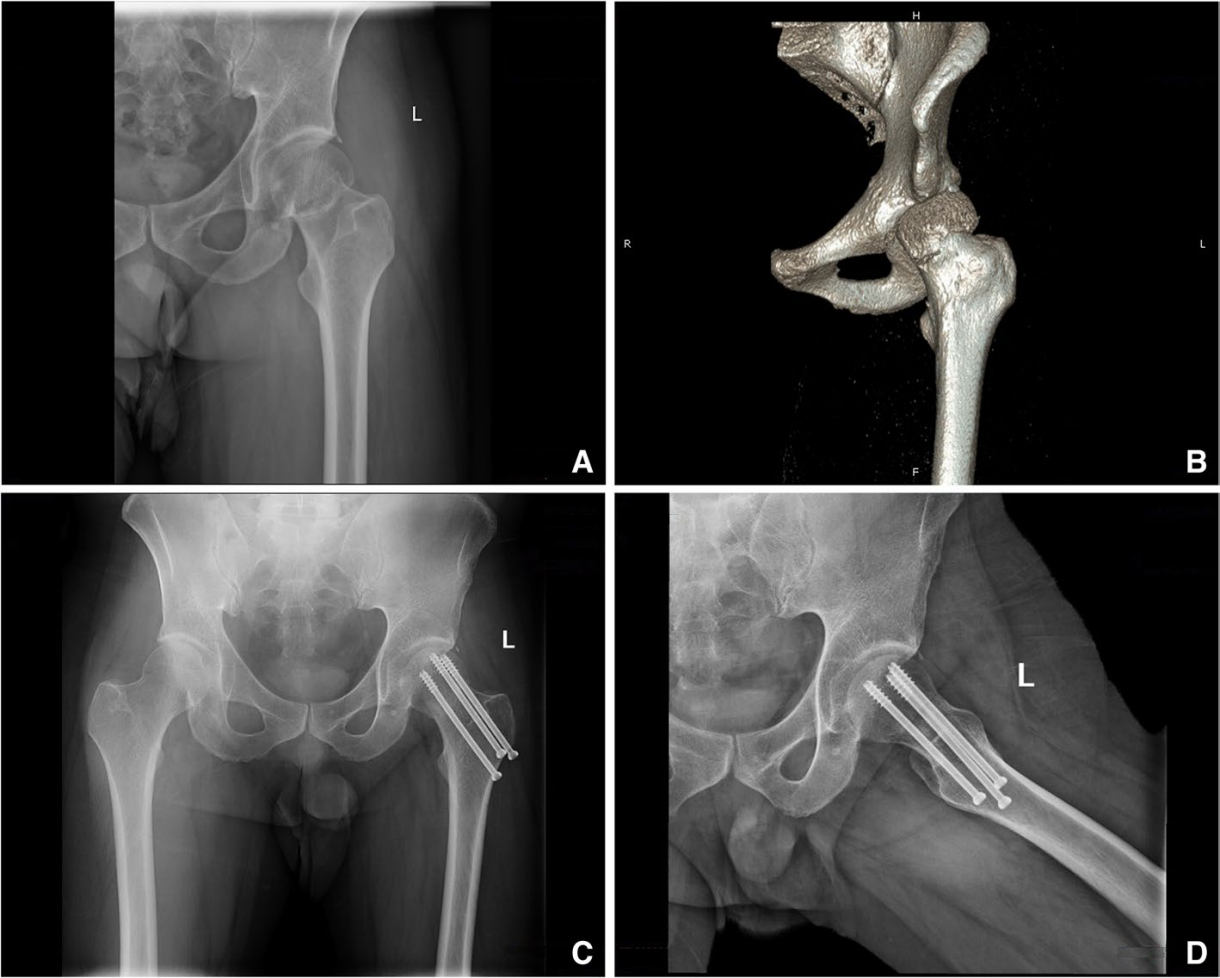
Preoperative X-ray and three-dimensional CT image of 39 years male with femoral neck fracture (A, B). X-ray image of this patient undertaken femoral neck cannulated screws with the laser guiding navigation device, and the fracture healed after operation 3 months later (C, D)
